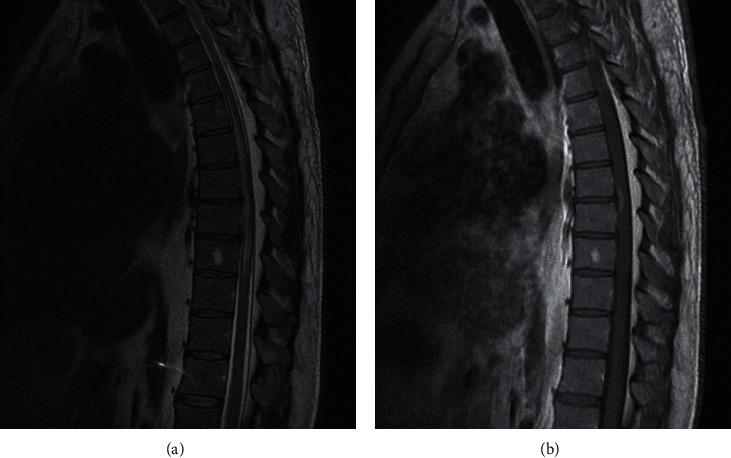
(a) Sagittal T2 MRI of dorsal spine showing high T2 signal with cord edema from T4 to T9 levels with thickened epidural fat tissue posteriorly. (b) Sagittal T1 MRI with gadolinium of dorsal spine showing an enhancing lesion at T7-T8 level.
